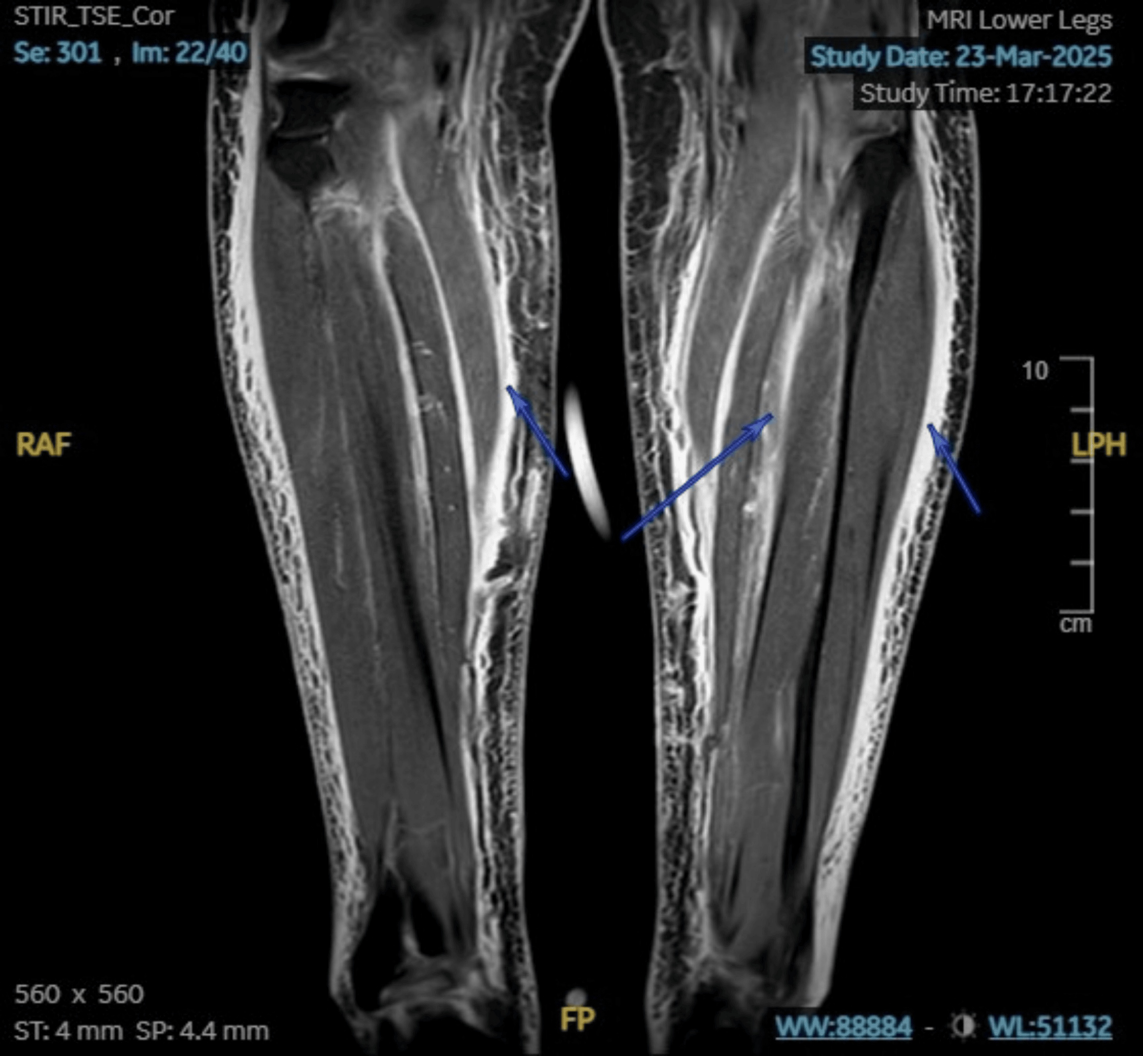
Coronal short tau inversion recovery (STIR) view of the MRI of the lower limbs.
